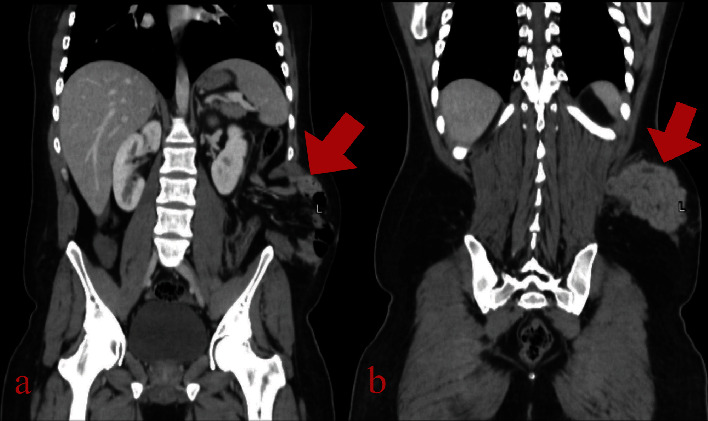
CT abdomen-pelvis imaging: (a, b) coronal slices, demonstrating the TAWH containing both small and large bowels.
